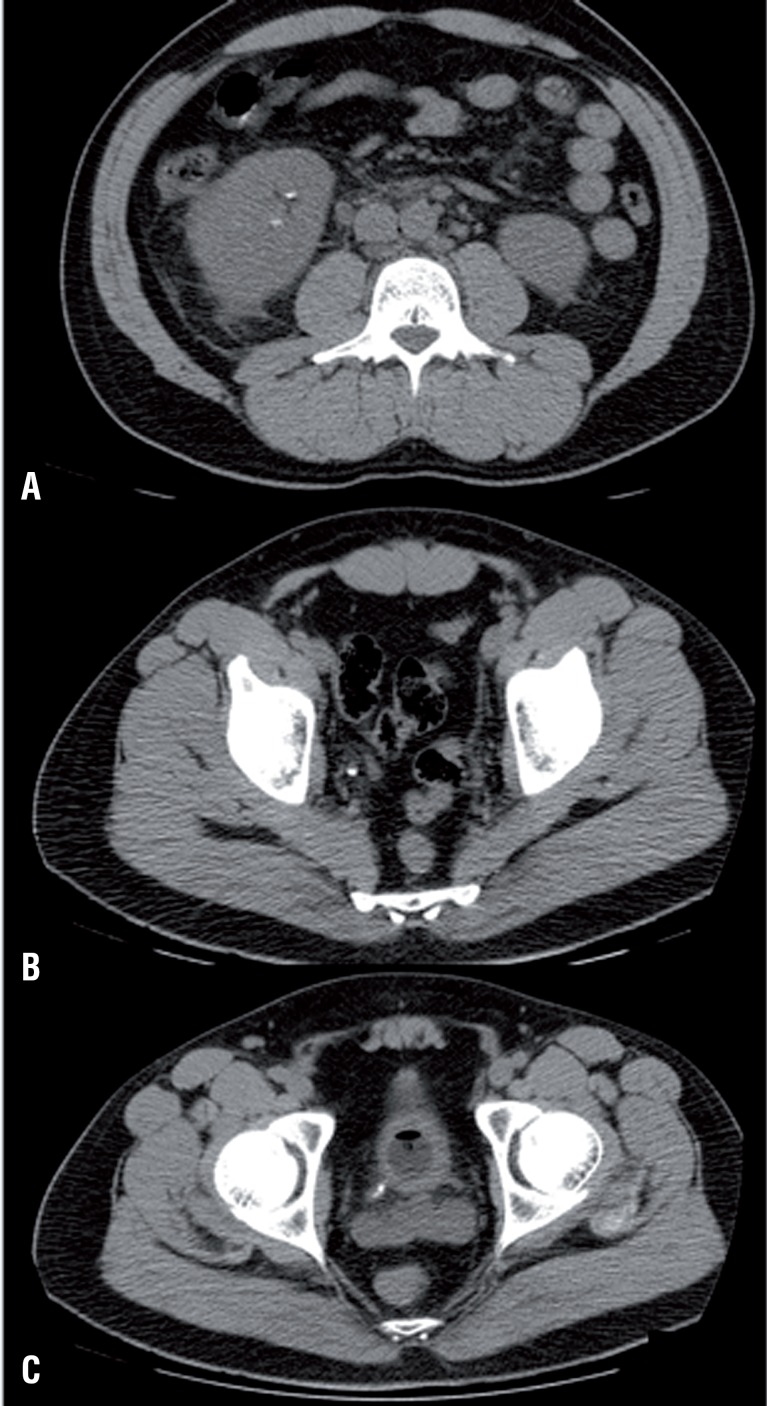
CT at the admission. Residual stone fragments in the lower calcyx (a), and in the lower tract of the ureter (b and c).
